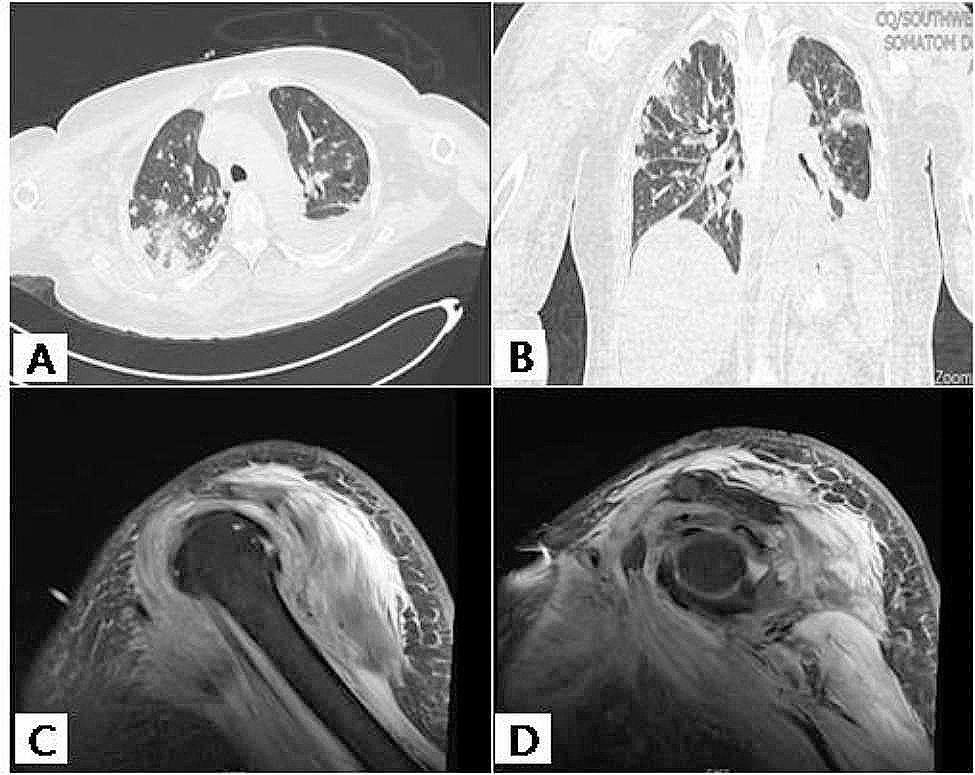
A-B: Chest CT images upon admission, revealing multiple lung lesions, coarse marginal nodules, atelectasis in the dorsal lobe of both lower lungs, pleural effusion on the left side, and minimal effusion in the right pleural cavity. C-D: Enhanced magnetic resonance imaging of the right shoulder, revealing periglenohumeral soft tissue edema and concurrent formation of multiple intermuscular abscesses
